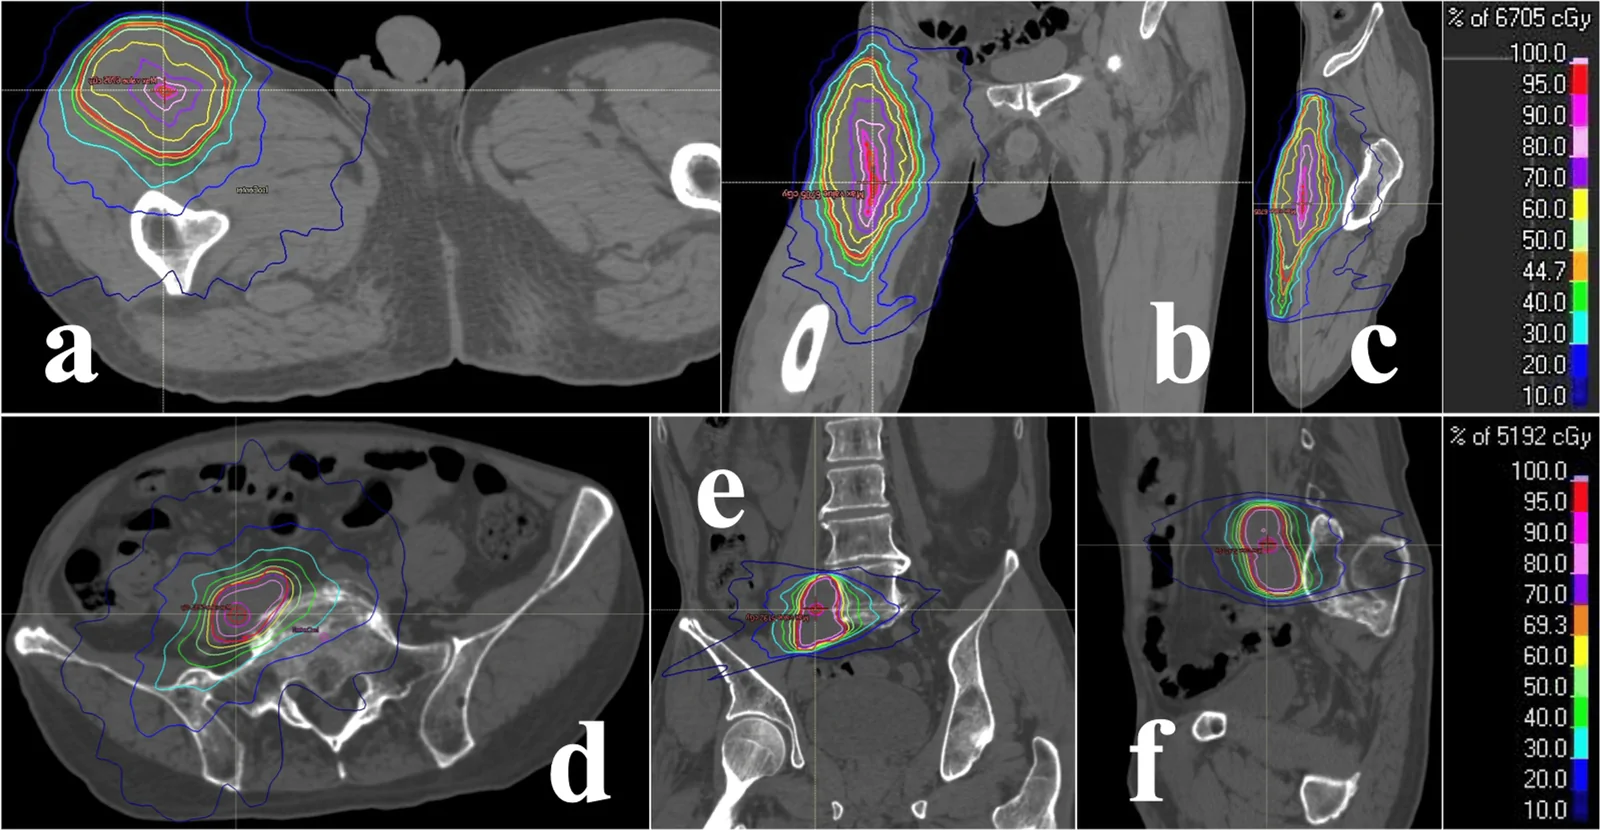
Planned dose distribution of SBRT. (a-c) Metastatic lesion in the right thigh. Isodose lines are shown relative to the maximum dose (100% = 67.05 Gy) on CT: (a) axial, (b) coronal, and (c) sagittal views. Irradiation time is 324 seconds per fraction via three arcs at O-ring angles of 0°, 15°, and 345° using the OXRAY system. (d-f) Metastatic lesion in the right abdomen. Isodose lines are shown relative to the maximum dose (100% = 51.92 Gy) on CT: (d) axial, (e) coronal, and (f) sagittal views. Irradiation time is 293 seconds per fraction via three arcs at O-ring angles of 0°, 20°, and 340° using the OXRAY system. SBRT, stereotactic body radiotherapy; CT, computed tomography.
